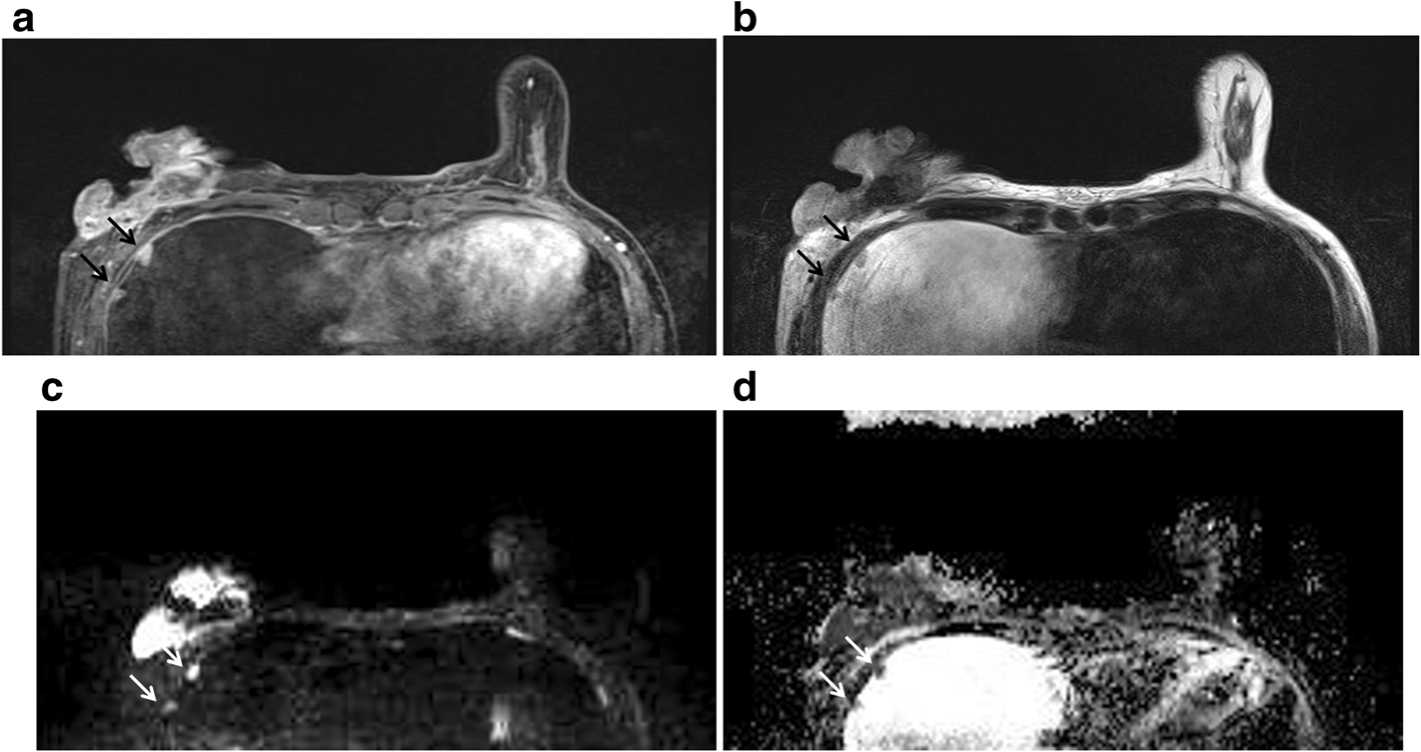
Multiple pleural metastases. Pre-operative breast MRI of a 46-year-old female patient with confirmed right breast cancer on biopsy. Multiple pleural nodules (*arrows*) showing contrast enhancement (a), isointense on T2WI (b), and definite diffusion restriction (c, d). These lesions were confirmed as pleural metastases in follow-up PET/CT and chest CT
